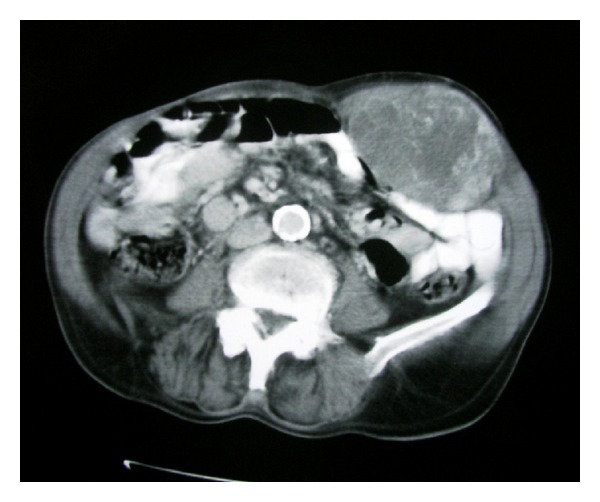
The abdominal CT demonstrates a 11 × 7 cm mass in diameter arisen from the rectus abdominis muscle.
